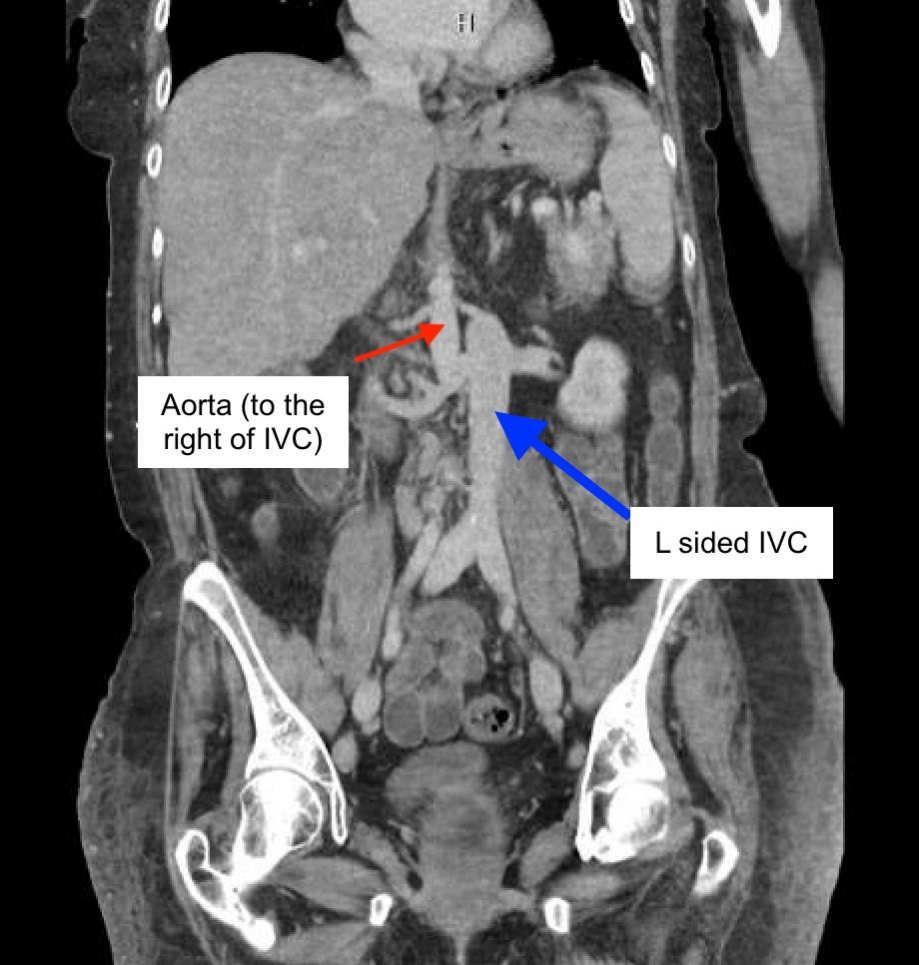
L sided IVC (blue arrow) with bilateral renal vein insertion. Aorta on the R side (red arrow). (CT abdomen/pelvis w/contrast - coronal view).
